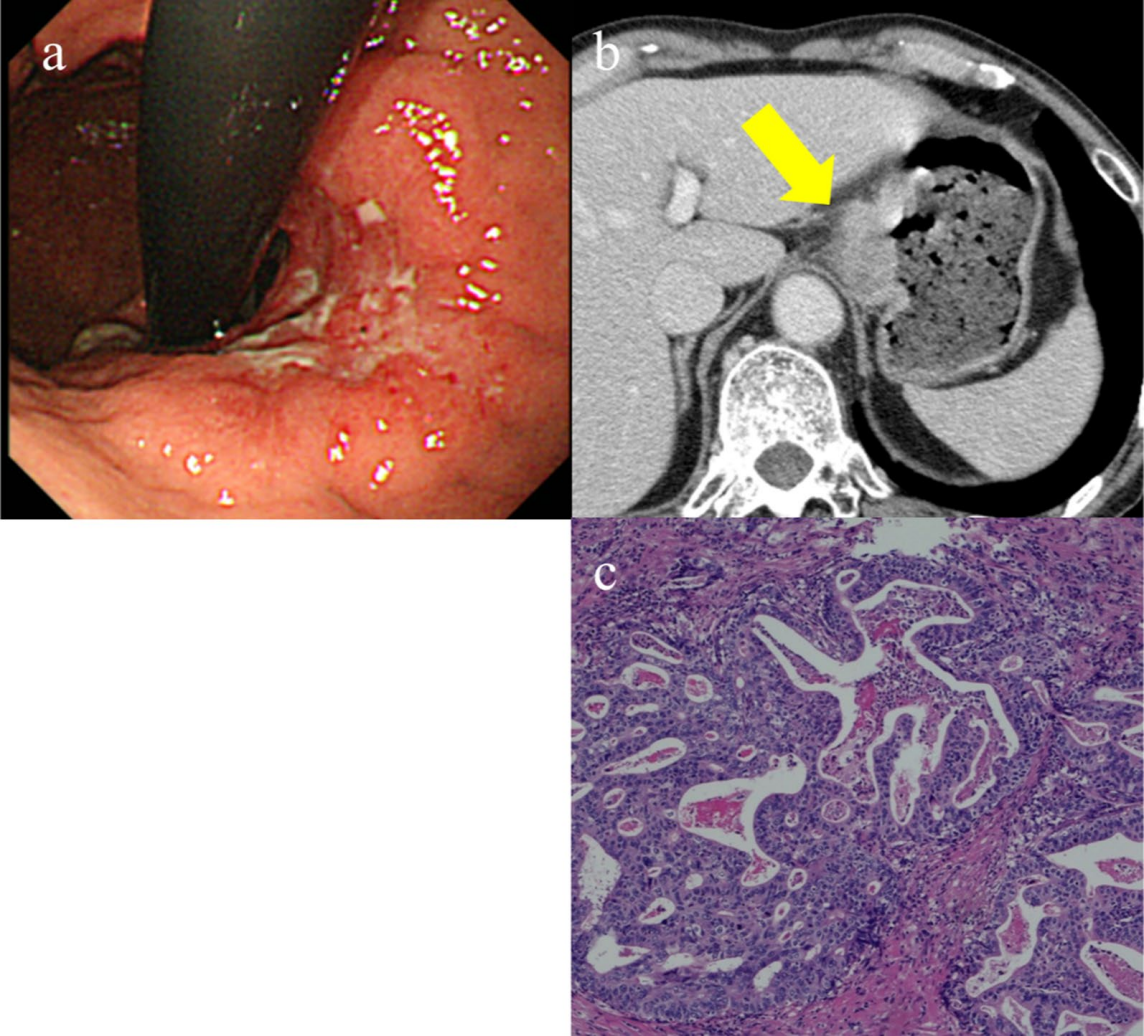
Computed tomography (CT) scan and pathological findings of the primary gastric cancer. a Upper endoscopy demonstrated circumferential ulcerated lesion at the cardia. b CT scan showing wall thickening at the lesser curvature side of the upper gastric body. c Pathological examination reveals a moderately differentiated adenocarcinoma
